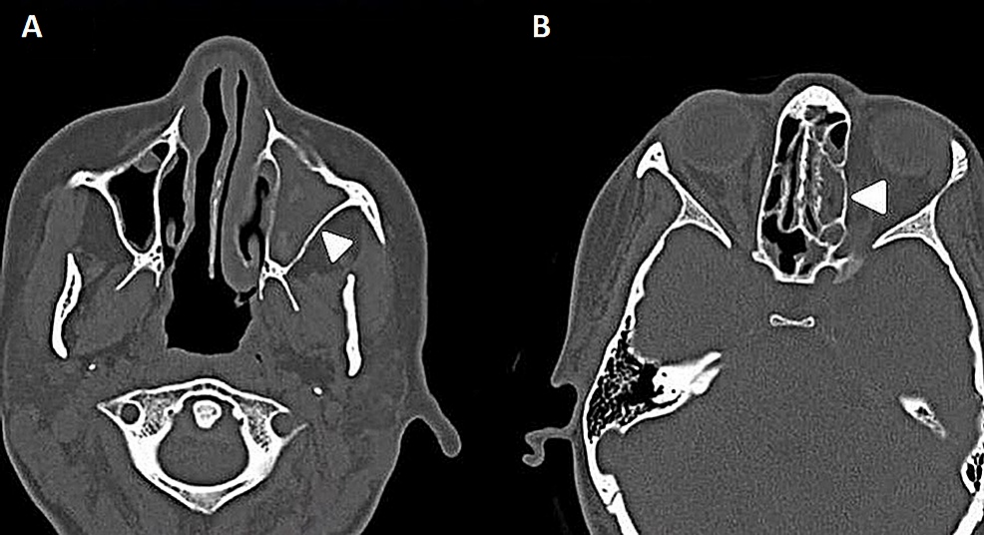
Pre-treatment CT scans showing persistent partial opacification of the paranasal sinuses with high internal densities. (A) Almost complete opacification of the left maxillary sinus prior to medication use (arrowhead); (B) mucosal thickening of ethmoid sinuses more marked on the left side prior to medication use (arrowhead).
